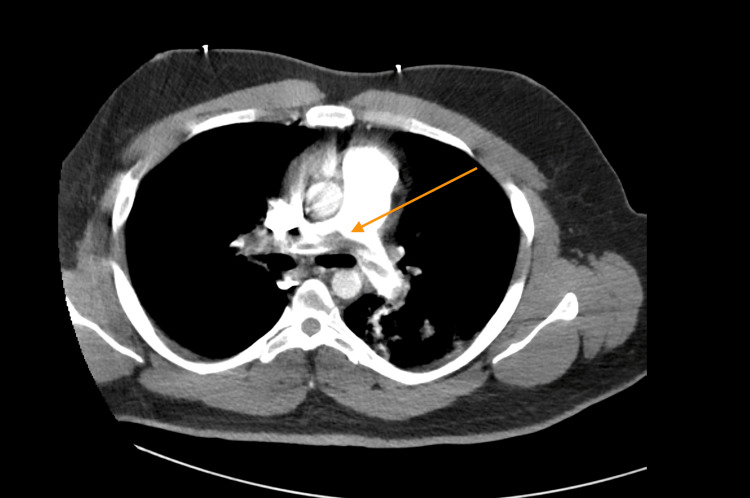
CT angiogram showing saddle embolus in pulmonary arteries
